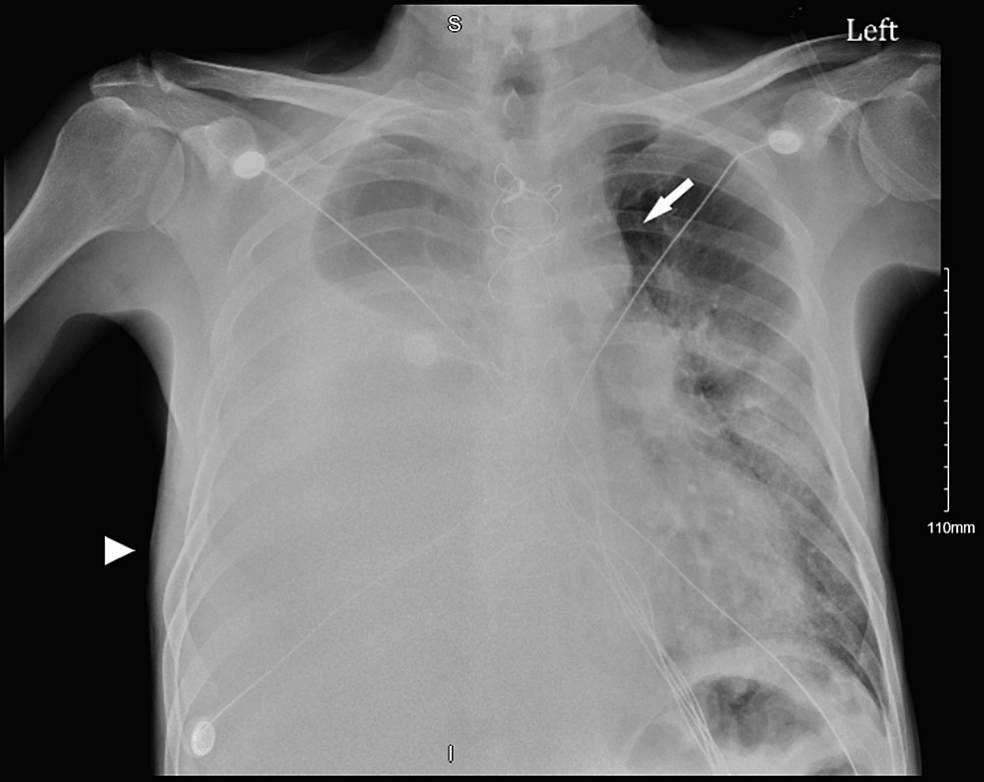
Chest radiograph. Chest radiograph depicting a large right pleural effusion causing opacification of the right hemithorax (arrowhead), with an associated shift of the heart and mediastinal structures into the left hemithorax (arrow).
